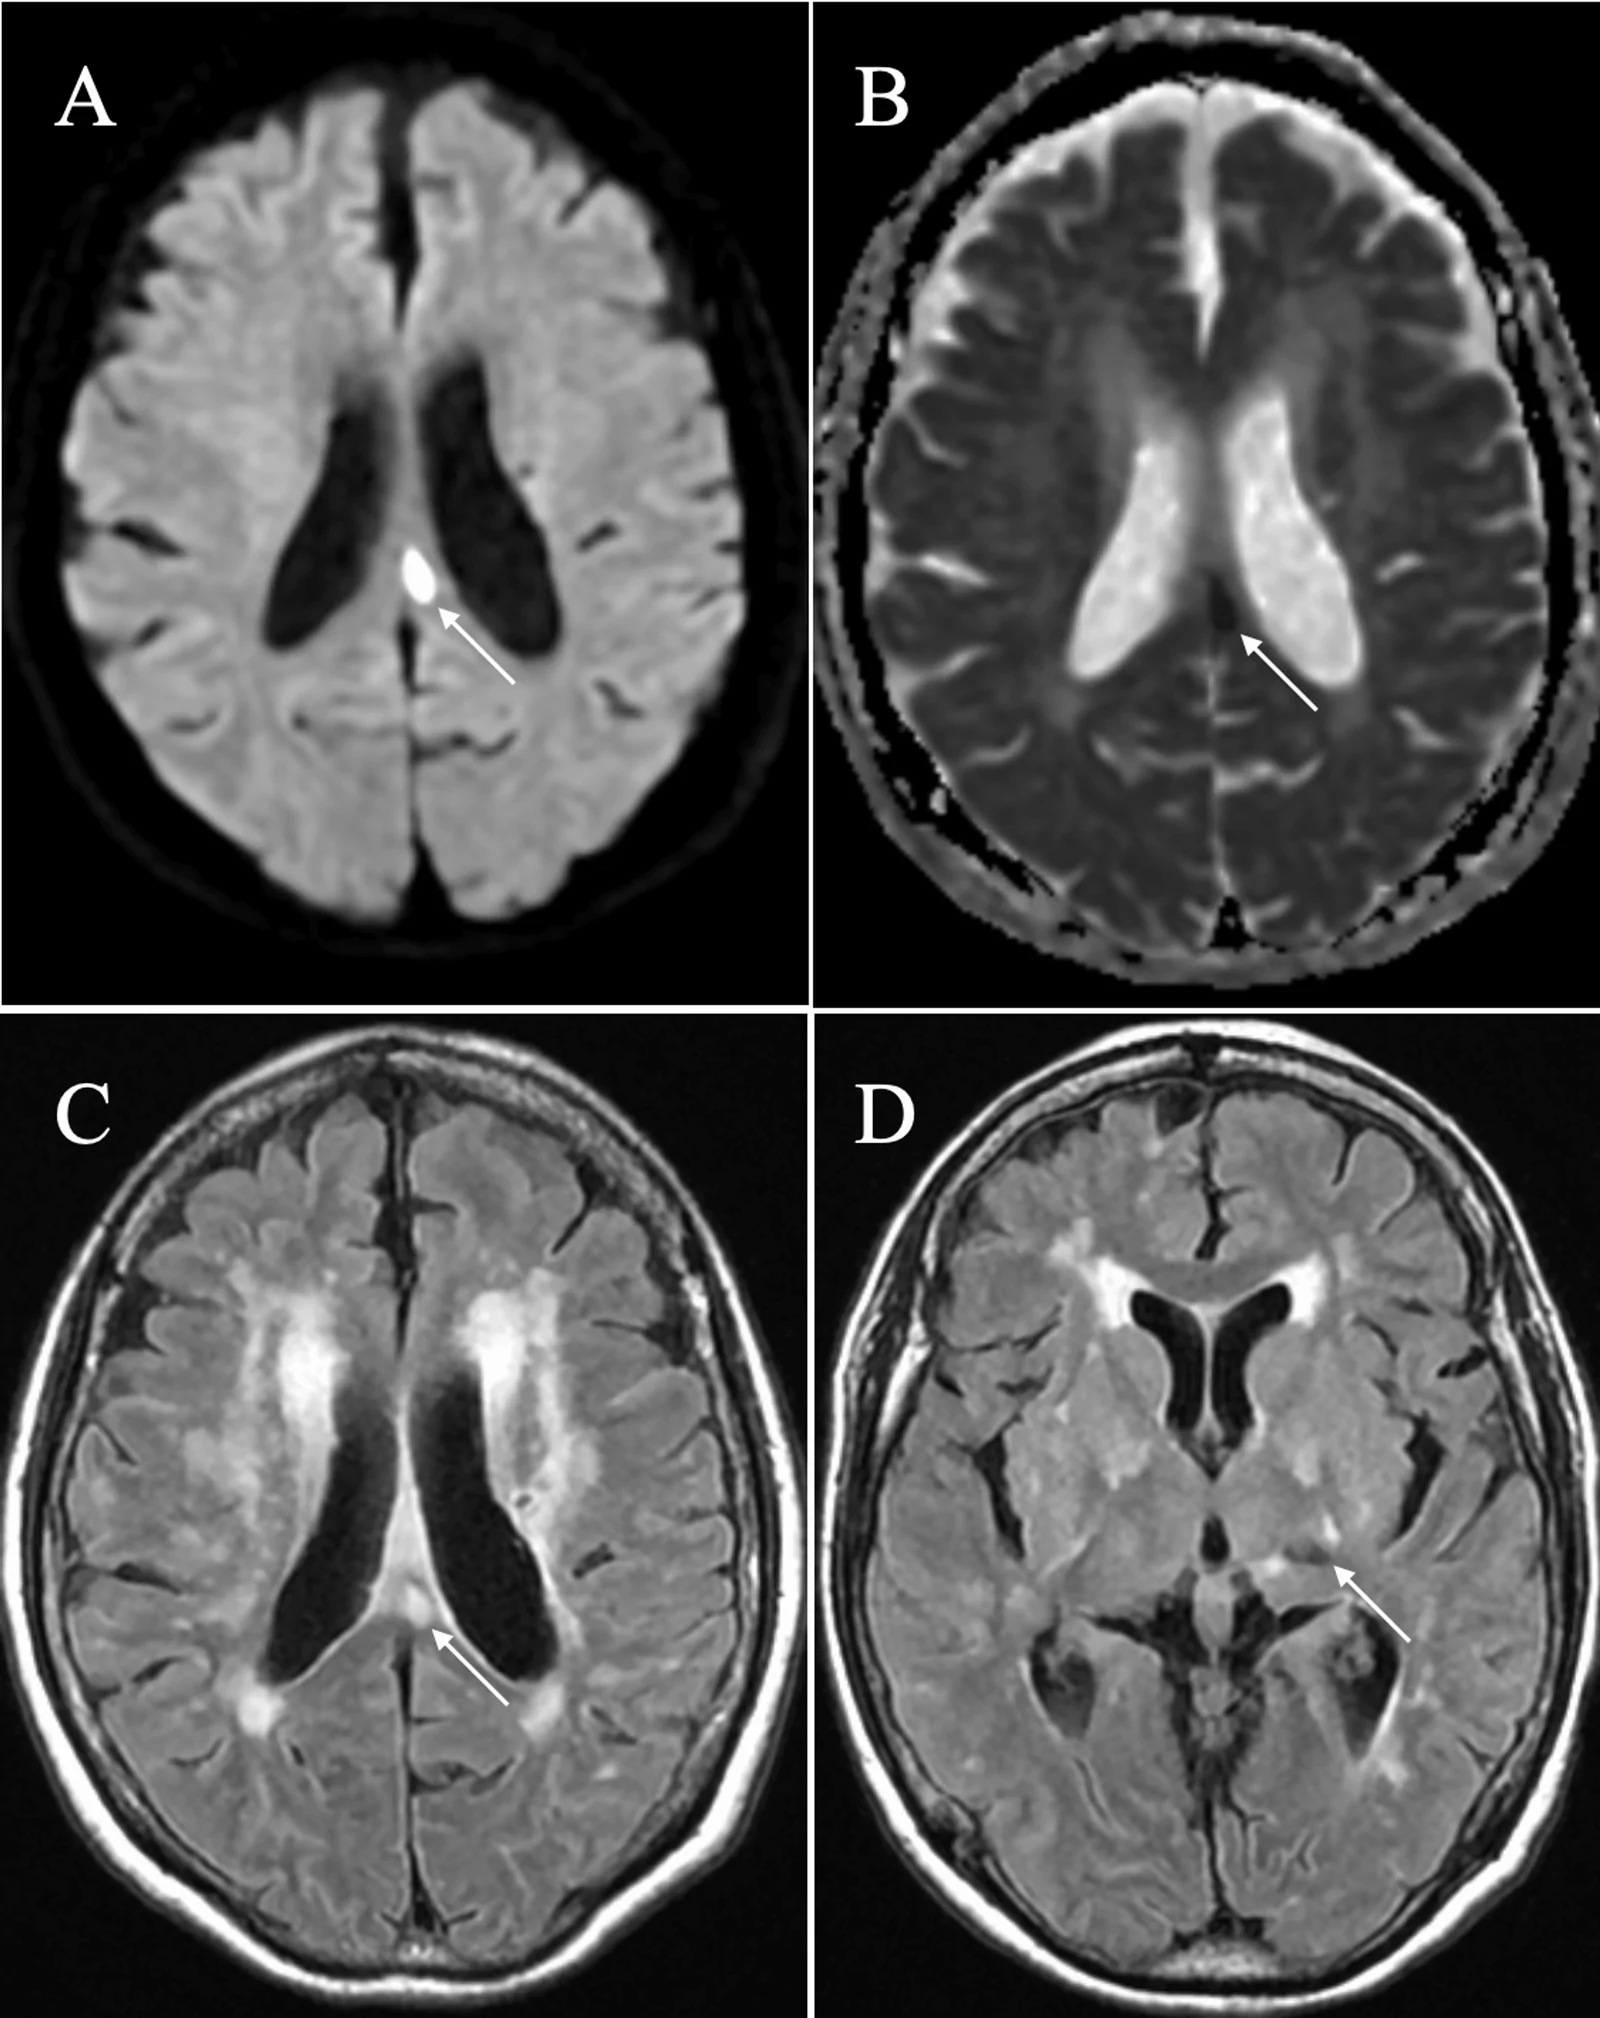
Brain MRI findings MRI showed a lesion in the splenium of the corpus callosum, showing high signals on diffusion-weighted images (A, arrow), low signals on apparent diffusion coefficient images (B, arrow), and high signals on fluid-attenuated inversion recovery images (C, arrow). FLAIR also showed a lesion in the left thalamus suggestive of an old cerebral infarction (D, arrows) MRI: magnetic resonance imaging; FLAIR: fluid-attenuated inversion recovery
